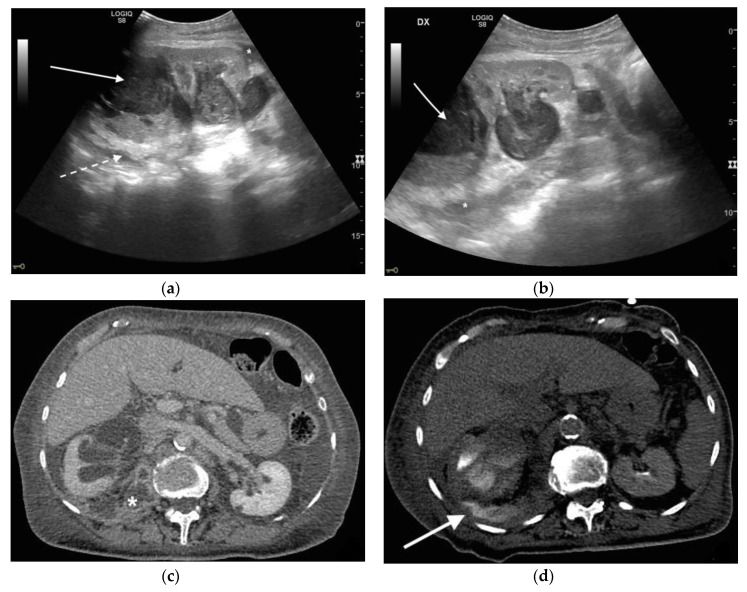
An 83 years old septic woman. US longitudinal (a,b) view of the right kidney showing high-grade hydronephrosis with the calyceal-pelvic system completely occupied by inhomogeneous echogenic debris (with arrow). Perirenal fascia is thickened and perirenal fat is hyperechogenic and inhomogeneous (dashed arrow). Fluid over-collection is appreciated around the kidney (*), and free fluid was appreciated in the abdomen. Complicated pyonephrosis with extrarenal extension was diagnosed. CT with intravenous contrast. Axial CT after intravenous contrast in parenchymal (c) and urography phase (d), showing hydronephrosis with parietal thickening of calyceal-pelvic system, multiple loculated over fluid collection in the perirenal (white arrow) space and abscess in iliopsoas muscle (*). In the urography phase, extravasation of urine into extrarenal fluid collections due to abscessualization of the calyceal-pelvic system.
